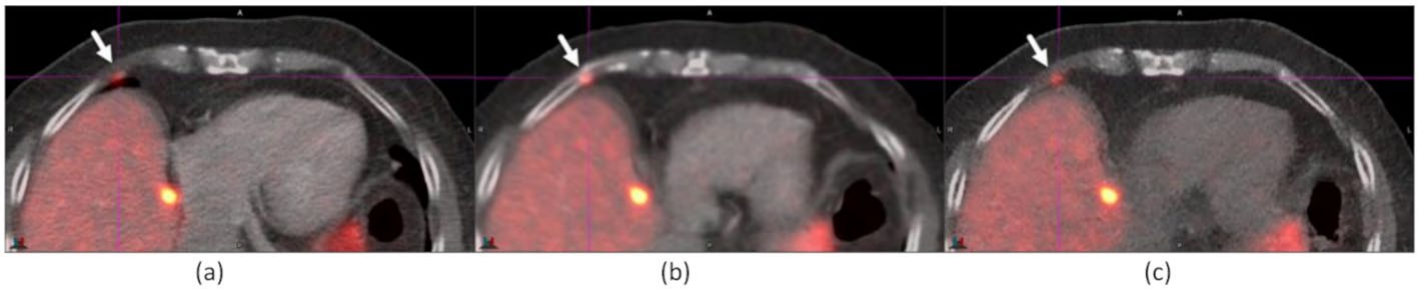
A 0.5-cm right supradiaphragmatic lymph node (indicated by an arrow) of a ^68^Ga-Dotatate study in baseline (a) and DDG CT (c) was mispositioned to the ribs in AIR (b). The SUV_max_ values were 8.62 for baseline, 9.19 for AIR, and 8.79 for DDG CT
